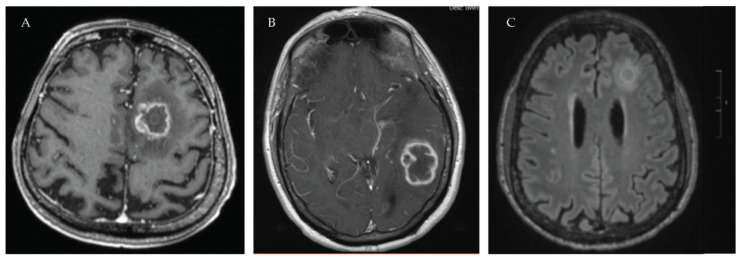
Central nervous system manifestations of IFI in patients receiving BTK inhibitors. IFIs involving the CNS have frequently been reported in patients receiving treatment with BTK inhibitors. The most common and earliest reported CNS infection was IA, by Ruchlemer et al. and Lionakis et al. However, other non-*Aspergillus* mold infections including *Scedosporium*, *Rhizomucor,* and *Lichthemia* sp. have been reported (Anastasopoulo et al.) as well as multiple cases of CNS cryptococcal infections (Chamilos et al.). (A) MRI brain of patient with *Scedosporium boydii* infection following treatment with ibrutinib for CLL. (B) MRI brain of patient with *Aspergillus fumigatus* brain abscess following treatment with ibrutinib for CLL. (C) MRI brain of patient with cryptococcoma following treatment with ibrutinib for Waldenstrom’s macroglobulinemia.
